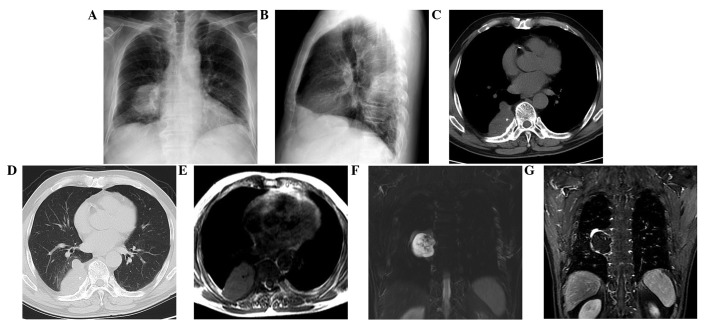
(A) Posteroanterior and (B) right lateral radiographs showing a round hyperdensity mass in the right lower lung lobe. (C and D) Non-contrast chest CT scan showing an oval mass with small calcifications in the posterior segment of the right lower lobe. The mass is located in the vicinity of the seventh rib and the T7 vertebral body. (E) T1-weighted axial MRI showing a right-lower-lobe mass with a signal intensity equal to that of the chest wall musculature. (F) T2-weighted coronal MRI showing that the signal intensity of the mass is similar to that of cerebrospinal fluid. (G) Contrast-enhanced T1-weighted coronal MRI showing that the mass is ring-like enhanced. CT, computed tomography; MRI, magnetic resonance imaging.
